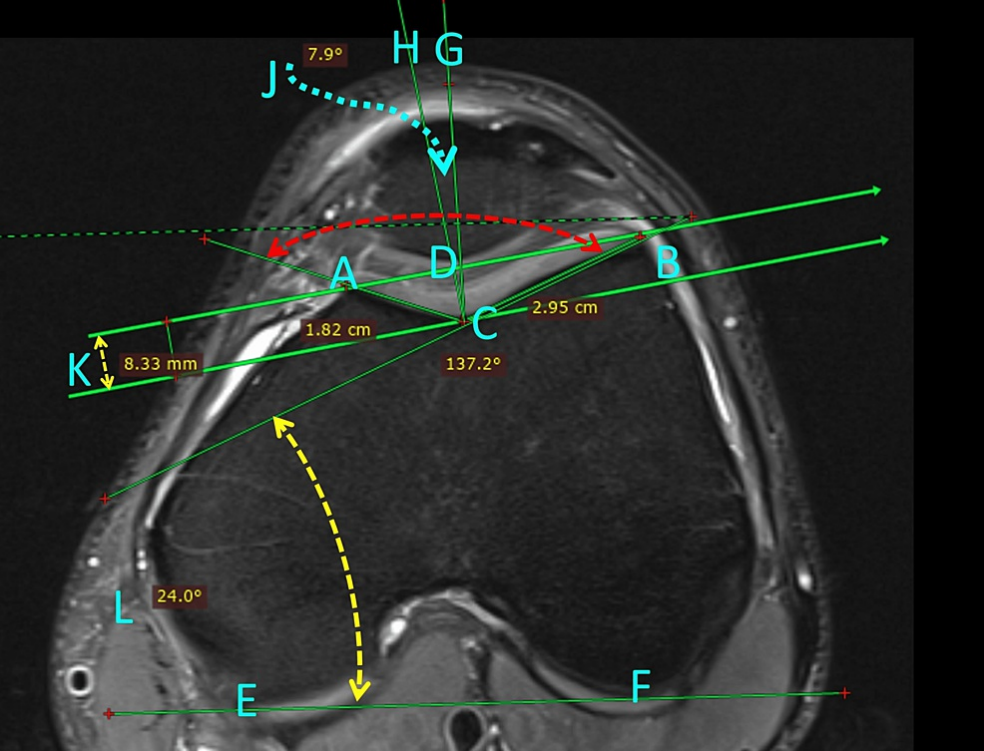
Measurements for TD Bony landmarks on axial MRI at the level of the fully shaped cartilaginous trochlea of the extended knee. (A) the highest point of the medial facet, (B) the highest point of the lateral facet, (C) the deepest point of the trochlear sulcus, (D) the apex of the median patellar ridge, (E) the posterior border of the medial femoral condyle, and (F) the posterior border of the lateral femoral condyle. The following measurements are made: AC: medial facet length, BC: lateral facet length, AC/BC: trochlear facet asymmetry, ACB: the sulcus angle (SA, red dotted line), CG: the bisector of the sulcus angle, DCG: the congruence angle (J, blue dotted line), the distance between the lines AB, and the line parallel through the point C is the sulcus/trochlear depth. The angle between the lines BC and EF is the lateral trochlear inclination (yellow dotted line). The RadiAnt-DICOM reader (Medixant, Poznań, Poland) was for the measurements. Figure created by Panagiotis Samelis.
